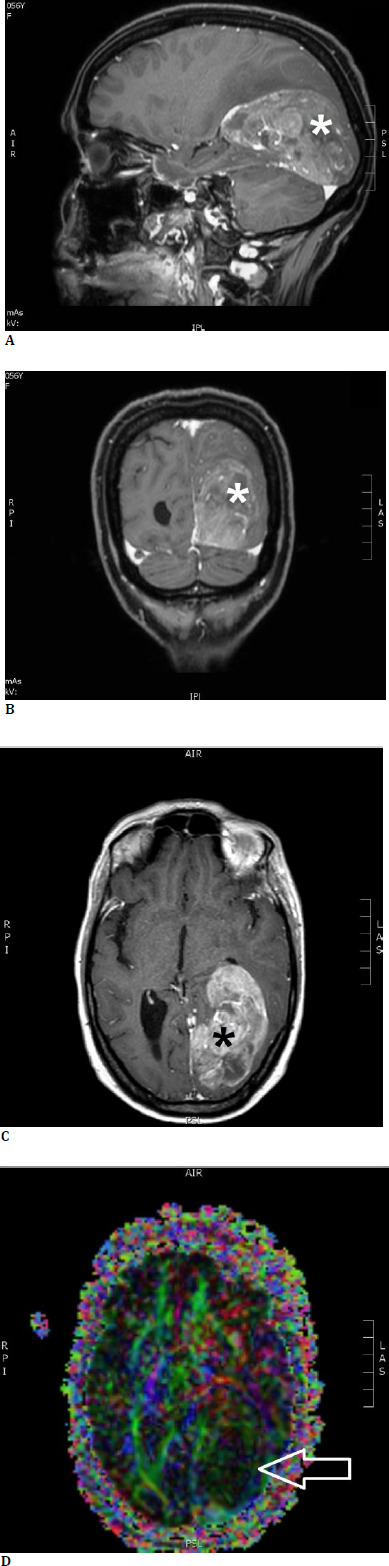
Brain MRI of our second patient, T1-weighted sequence with intravenous contrast, showing a giant left temporooccipital metastasis (*) from lung carcinoma. A. sagittal section; B. coronal section; C. transverse section; D. tractography showing distortion of the fibers of the left optic radiation (arrow)
